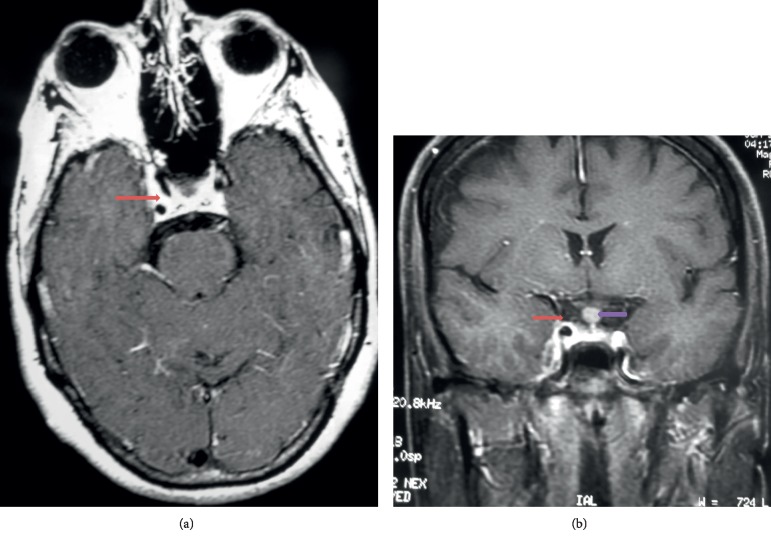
Magnetic resonance imaging after 23 years of mifepristone treatment. (a) Axial and (b) coronal sequences, in T1, after contrast, show significant reduction of the parasellar and dura mater expansive lesion (red arrow), with persisting thickening of the pituitary stem (purple arrow).
